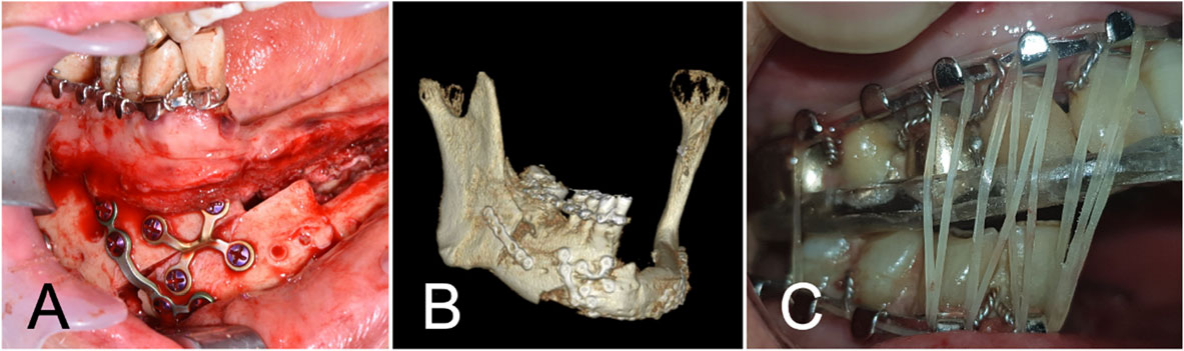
Secondary surgery for the reestablishment of occlusion and facial symmetry. a Sliding osteotomy at the anterior junction between FFF and #42 tooth on the mesial side. b 3D reconstruction image of CT scan after SSRO of the right mandible and sliding osteotomy of the anterior mandible. c Elastic intermaxillary fixation with occlusal wafer after restoration of normal occlusion
